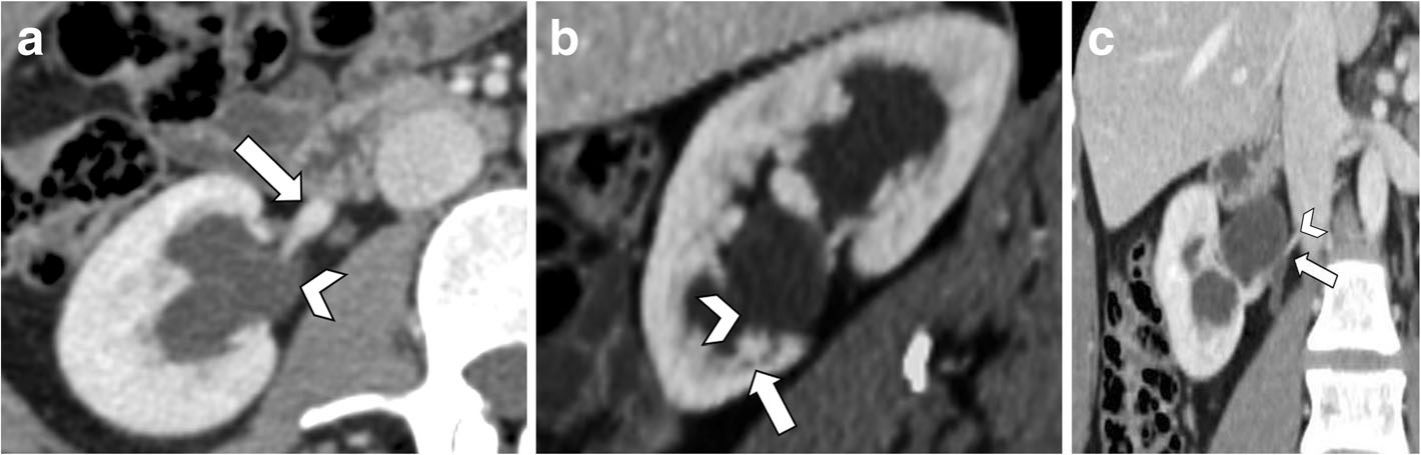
A 31-year-old woman with ureteropelvic junction obstruction. a Axial CT image shows pelvic dilatation (arrowhead) caused by ureteropelvic junction compression by lower pole segmental renal artery (arrow). b The image reconstructed through an oblique sagittal plane, known as “hilar clock-face view”, obtained orthogonal to the renal hilum, shows the relationship between the lower pole segmental renal artery (arrow) and the ureteropelvic junction (arrowhead). c Coronal CT image on the portal venous phase shows the ureteropelvic junction compressed (arrow) by lower pole segmental renal artery originating from the abdominal aorta (arrowhead)
